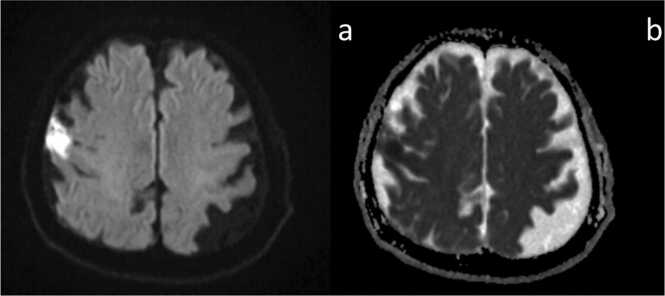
The repeat MRI (a, b: DWI) on the second day of admission. The diffusion-weighted image (a) demonstrates an area of marked high signal intensity in the subarachnoid space lateral to the right frontal lobe, accompanied by a slight decrease in the ADC value (b).
